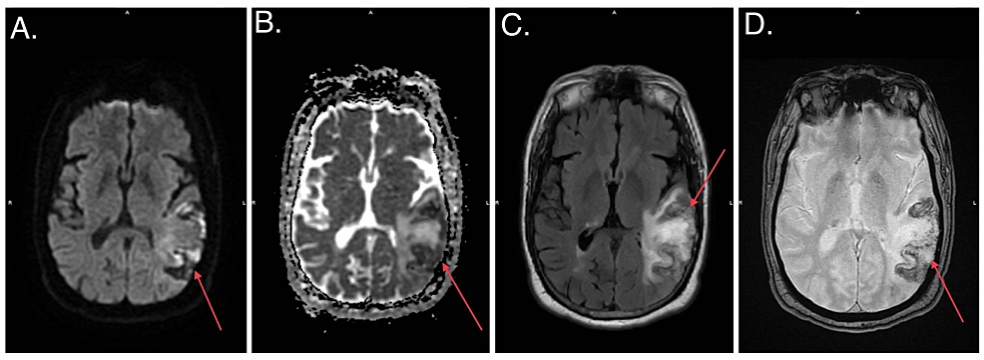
MRI showed an evolving hemorrhage in the left posterior parietal lobe and persistent multiple foci of abnormal signal intensity in the cerebral white matter (red arrows).
